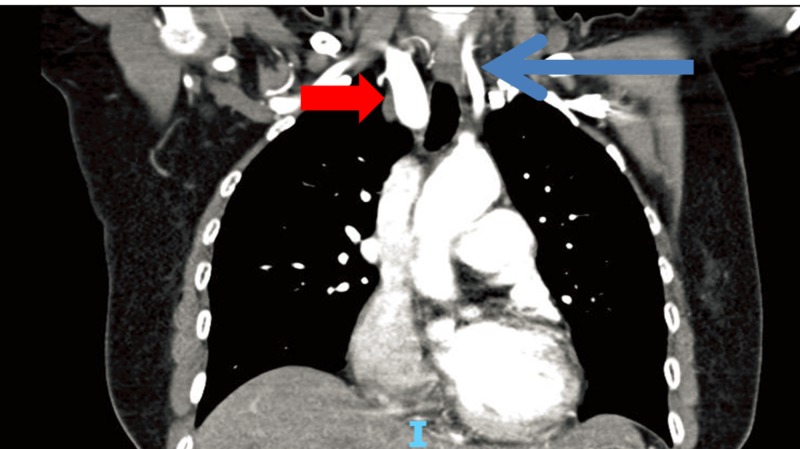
Computed tomography (CT) showing aneurysmal dilatation of the right subclavian artery (red arrow) as compared to the left subclavian artery (blue arrow)
